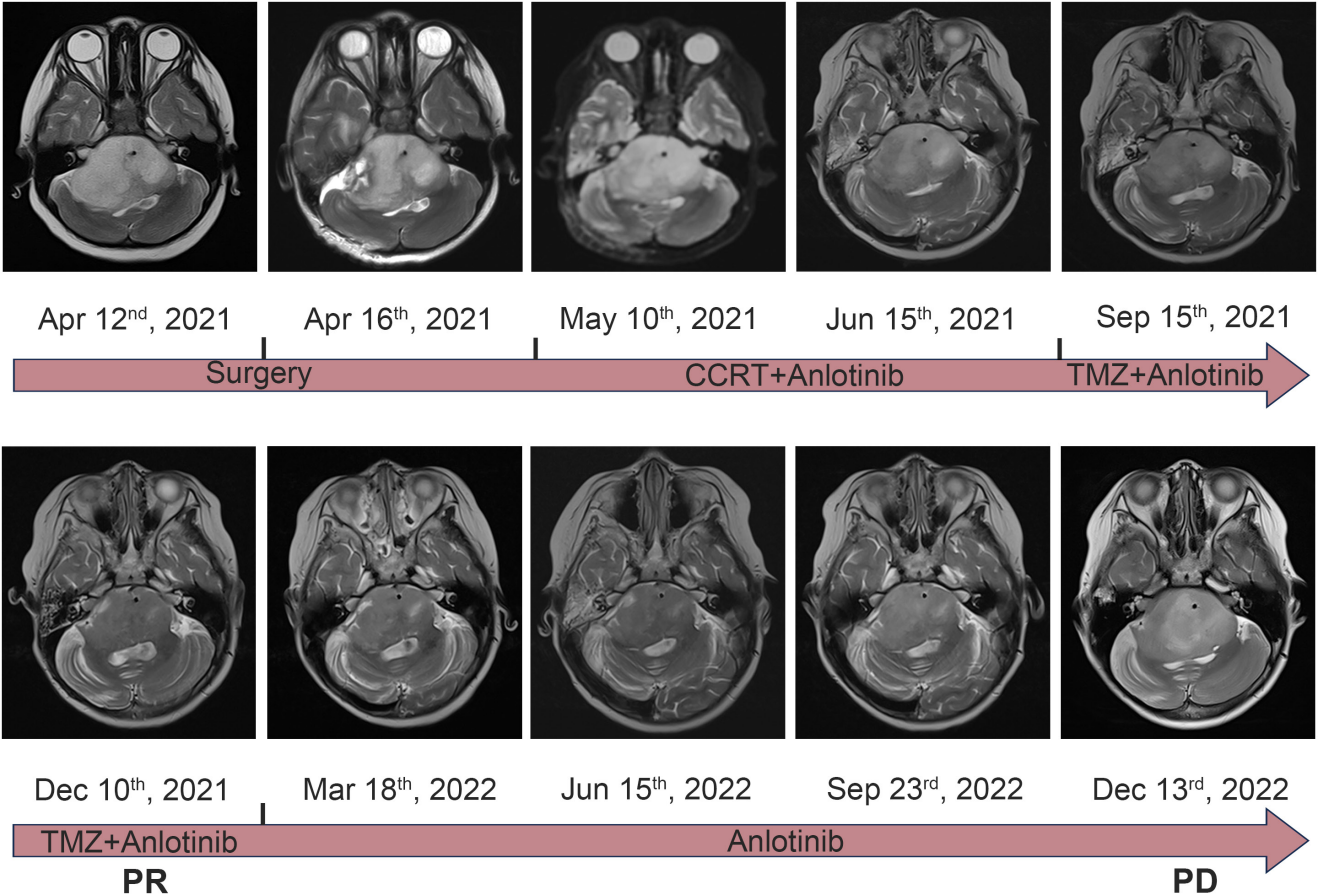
MRI changes of a representative case of DIPG treated with anlotinib. The best response achieved was PR, with a PFS of 20.3 months. CCRT, concurrent chemoradiotherapy; DMG, diffuse midline glioma; MRI, magnetic resonance imaging; PD, progressive disease; PR, partial remission; TMZ, temozolomide.
